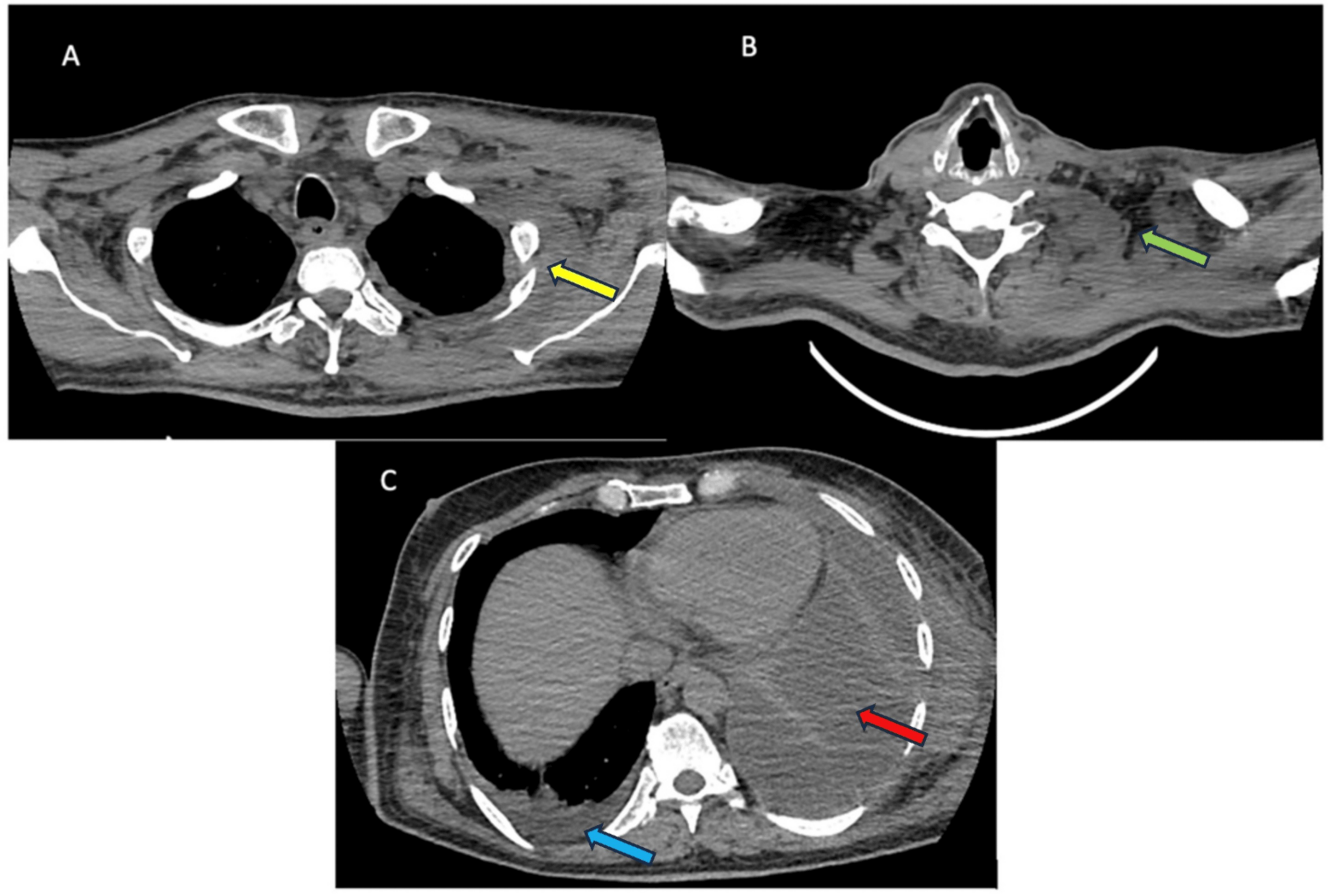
CECT chest and neck Demonstration of left-sided loculated effusion (red arrow) (c) with extension into the upper lateral chest wall (yellow arrow) (a), necrotic left cervical lymphadenopathy (green arrow) (b) with mild right-sided pleural effusion (blue arrow) (c). CECT: Contrast-enhanced computed tomography
